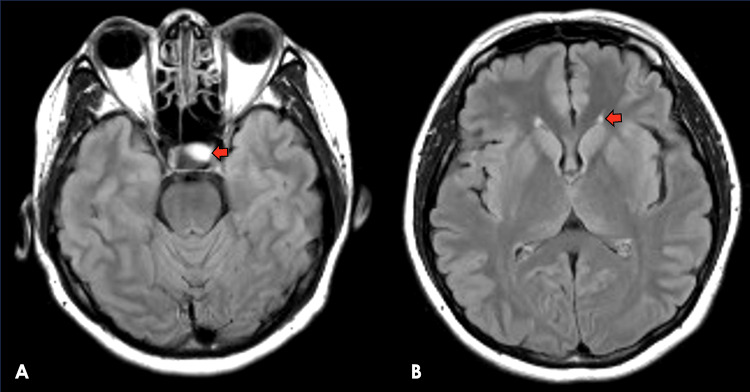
Axial fluid-attenuated inversion recovery (FLAIR) MRI of the brain demonstrates a known left-sided pituitary macroadenoma (A, red arrow) and a few chronic small-vessel ischemic white matter changes (B, red arrow). No imaging features suggestive of central nervous system demyelination are identified.
